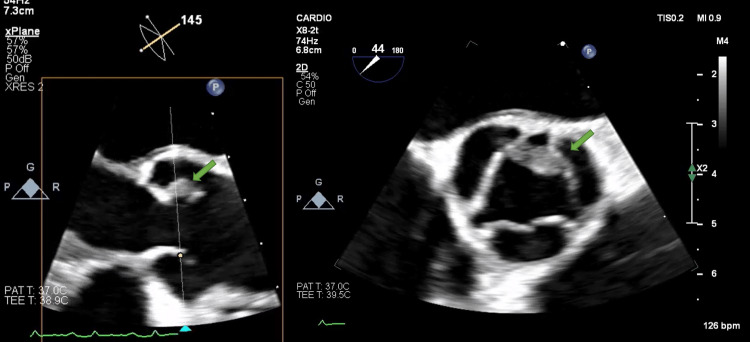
Biplanar TOE showing an 8 mm echogenic mass between the coronary and noncoronary cusps of the aortic valve (indicated by the green arrows) TOE: transesophageal echocardiography
